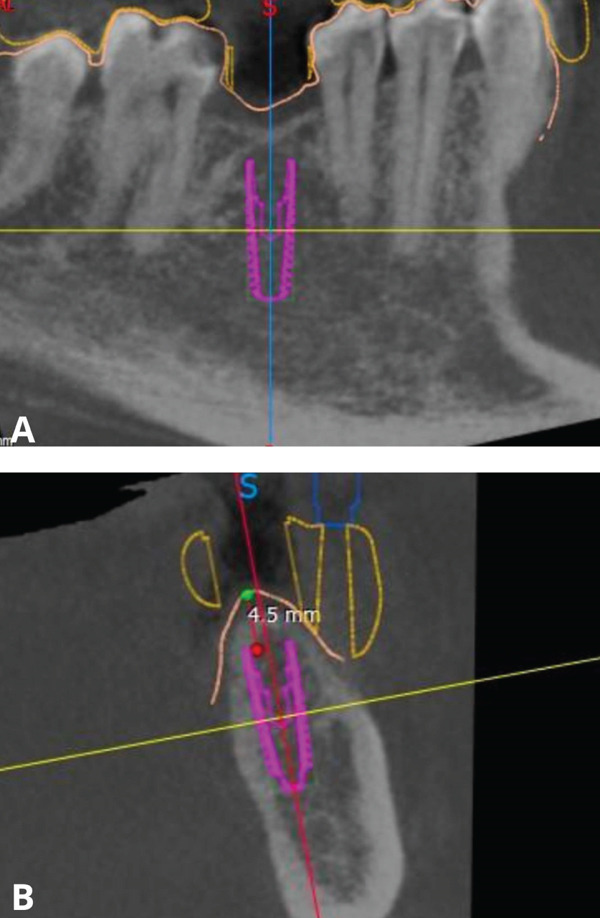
Implant placement planning based on CBCT. (A) Coronal view and (B) sagittal view.
